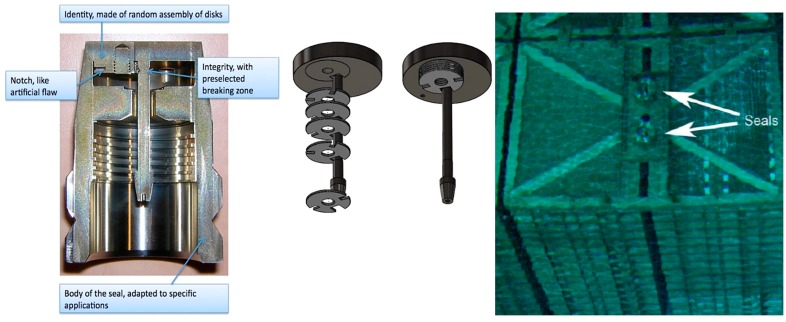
The Ultrasonic Sealing Bolt (USSB) structure and its application on underwater storage casks for nuclear items.
